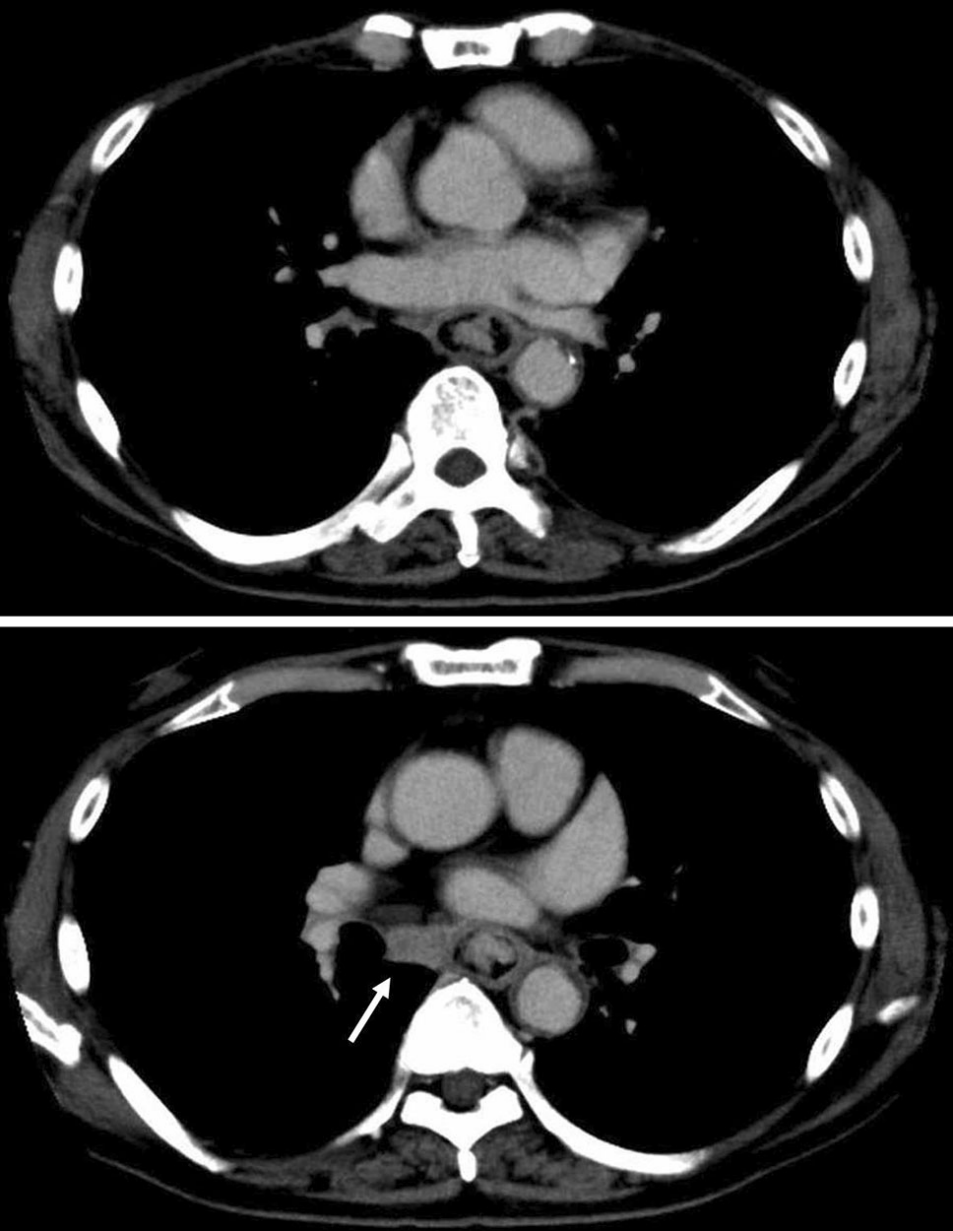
Contrast-enhanced thoracoabdominal CT reveals a tumor in the mid-esophagus, and an enlarged lymph node adjacent to the right main bronchus
